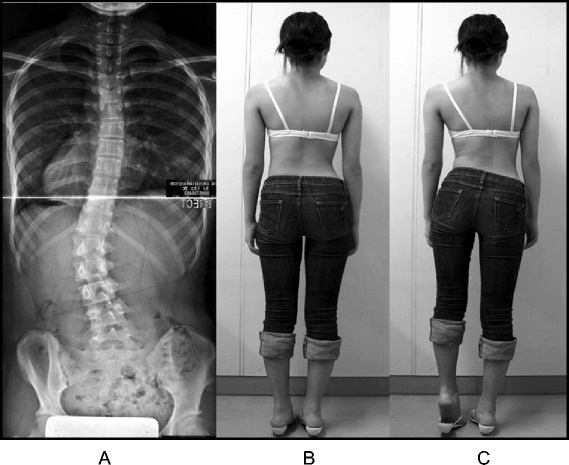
(a, b, c): “Hitch” exercise. A patient with a left thoracolumbar scoliosis curve seen on radiograph (a) stands in a neutral position (b). She is instructed to transition into the “hitch” position (c) by lifting her left heel on the same side as the convexity of the curve while keeping her hip and knee straight. The “hitch” position reduces the asymmetry of the patient’s waistline (c)
